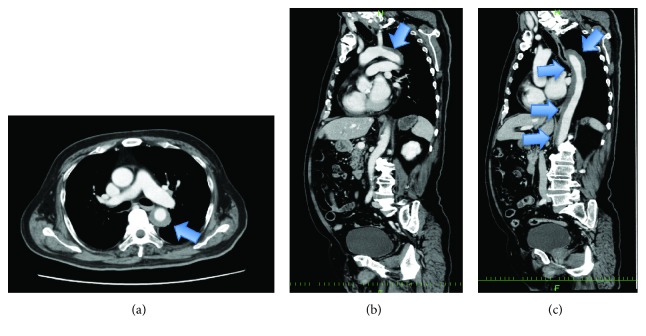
Case 2. Preoperative computed tomography: (a) axial image, (b) sagittal image, and (c) sagittal image in another slice. Intraluminal hematoma was observed (arrows).
